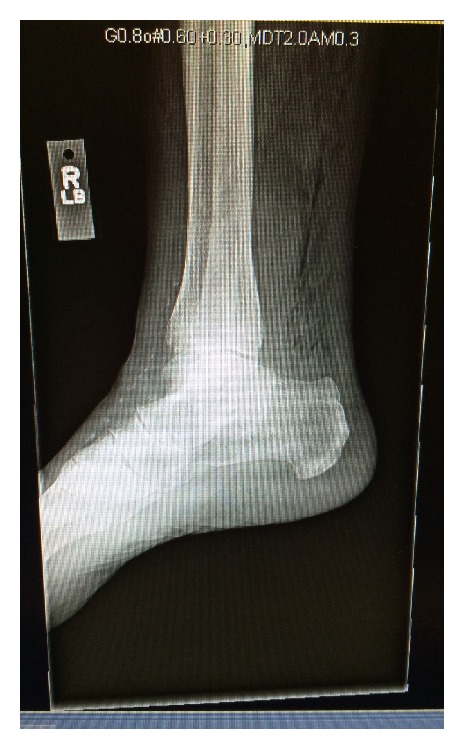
Radiograph of right ankle. There is diffuse subcutaneous air noted posteriorly to the tibia.
